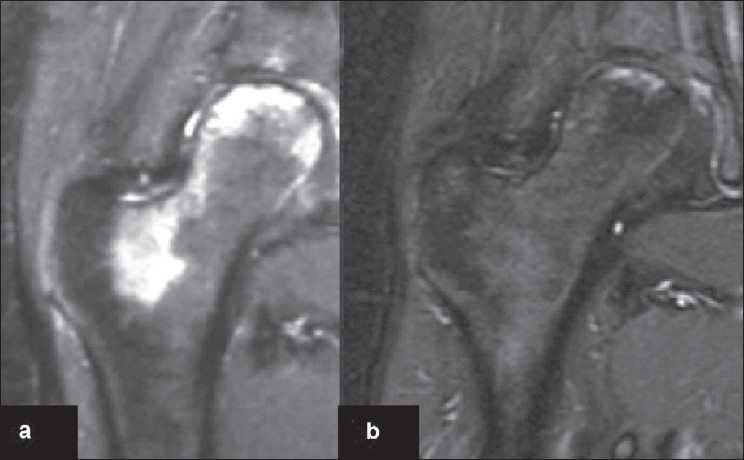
MRI of right hip (a) of 17 year old female shows edema with ficat stage II osteonecrosis shows bone edema. (b) MRI of the same hip after 6 months of stimulation and at the end of the treatment shows decreased bone edema and the patient had no pain.
